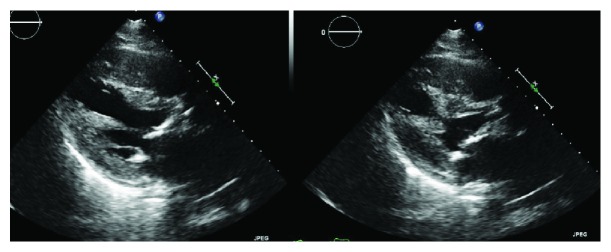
Transthoracic echocardiography with akinetic distal half of both left and right ventricles with resulting left ventricle outflow tract obstruction from the hyperkinetic basal myocardium.
